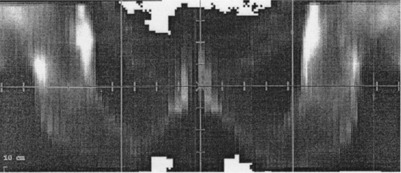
Digitally reconstructed radiograph from treatment CT, used to determine isocenter shift vs planning position.
